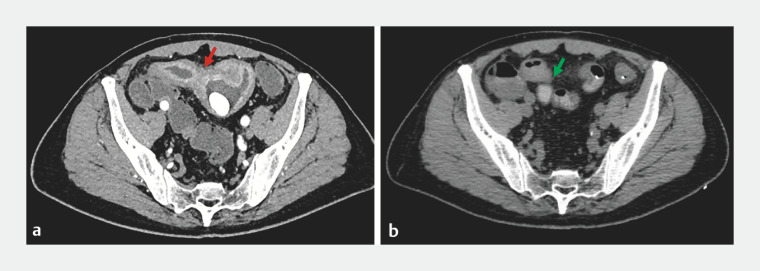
Computed tomography images of the distal ileum before (
